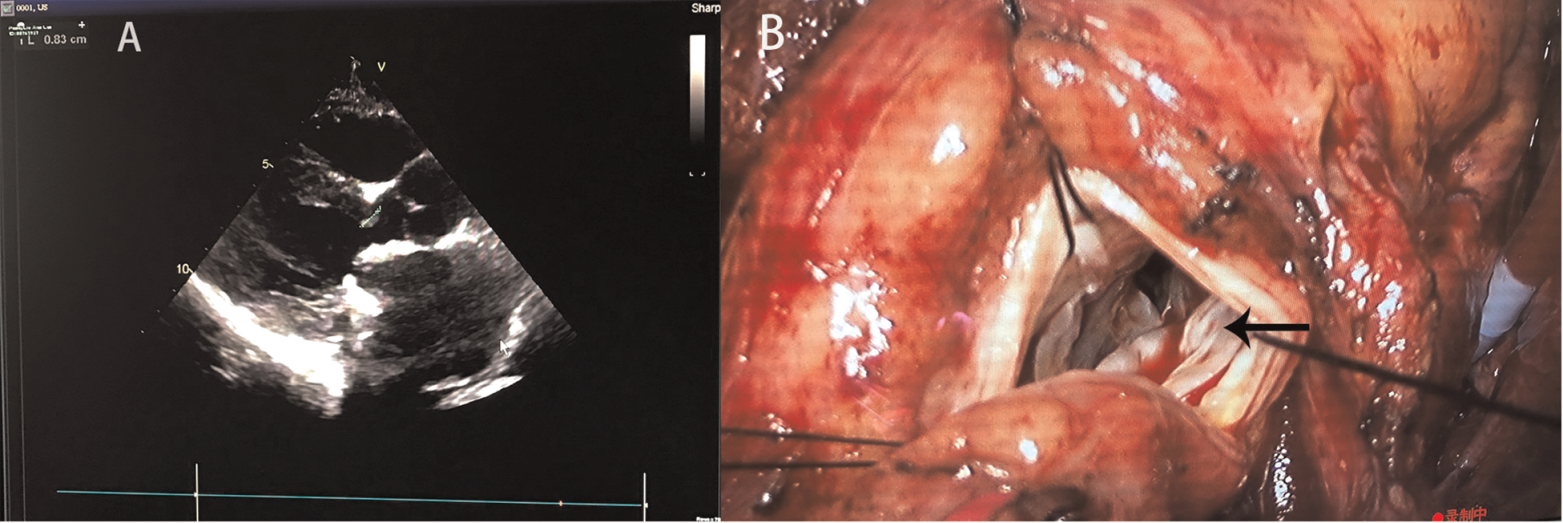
(A) Preoperative echocardiography showed and (B) intraoperative findings of Case 1. The black arrow indicates the protruding mitral annulus tissue.
